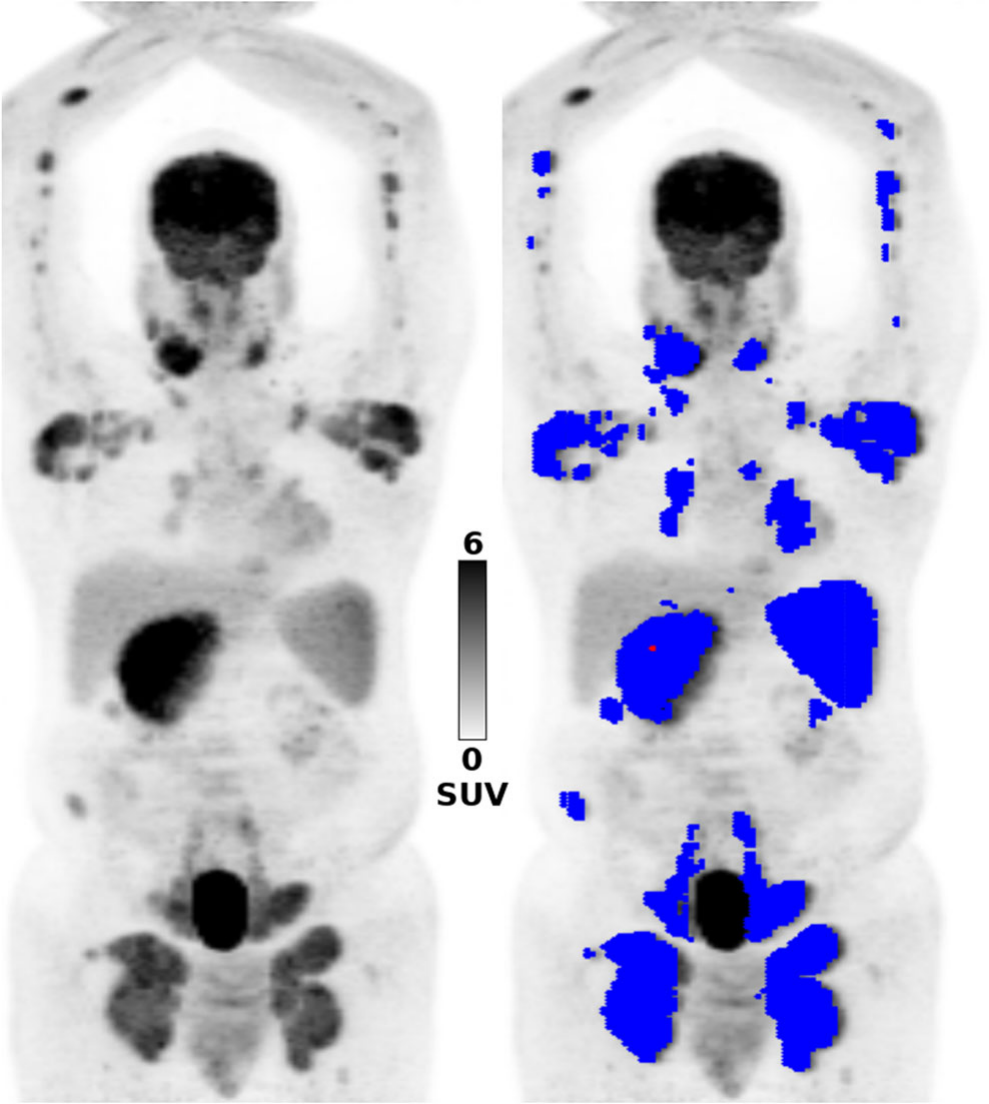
A 66-year-old patient with stage IV mantle cell lymphoma: *left* [^18^F]FDG PET maximum intensity projection image; *right* 3D radiomic analysis based on the total metabolic tumour volumes (*blue*) constructed using the previously recommended 41% SUVmax threshold; the SUVmax (*red dot*) was measured in the periportal nodal bulk
